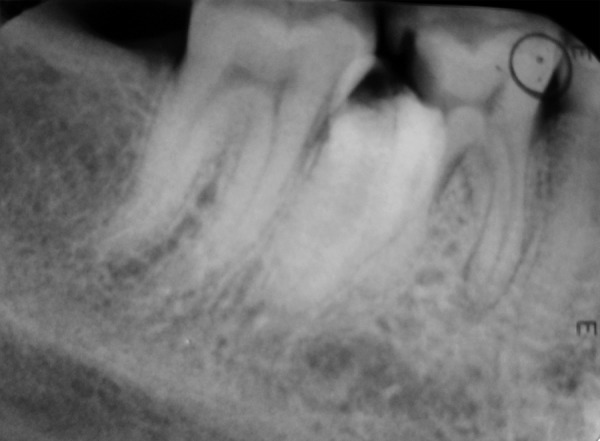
Intraoral radiograph showing paramolar (right side)
